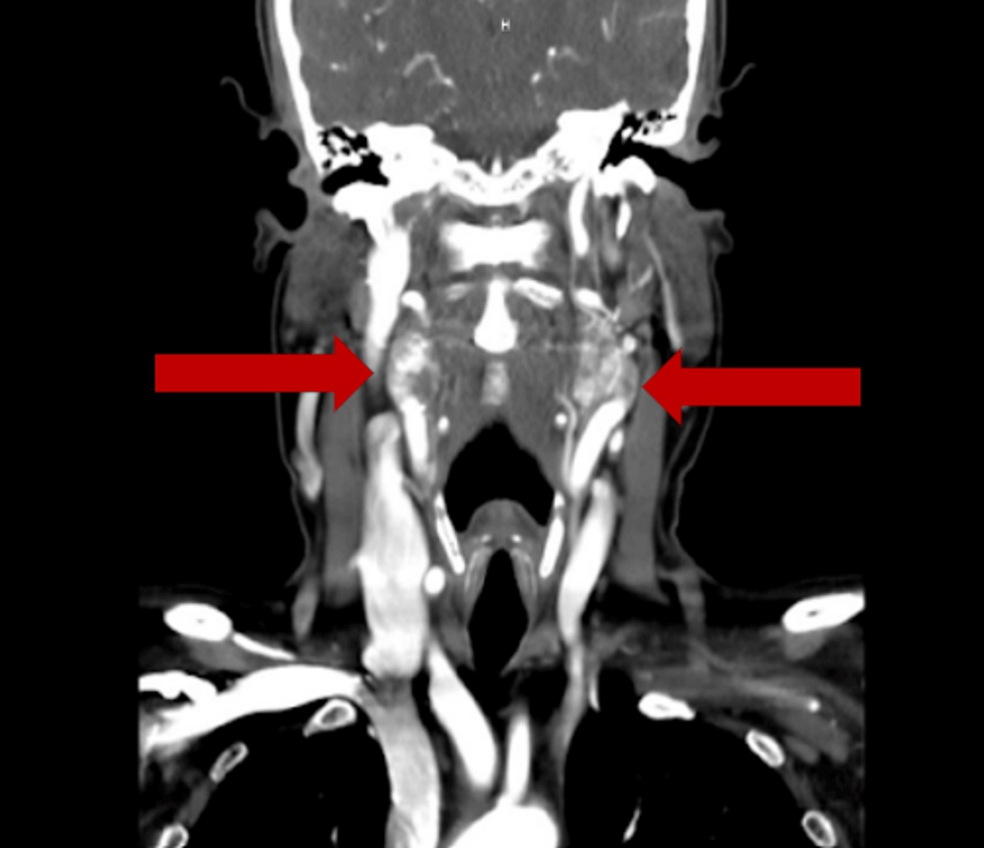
Coronal CT angiogram showing heterogenous enhancement of carotid body tumors bilaterally without significant vascular compromise (red arrows)
